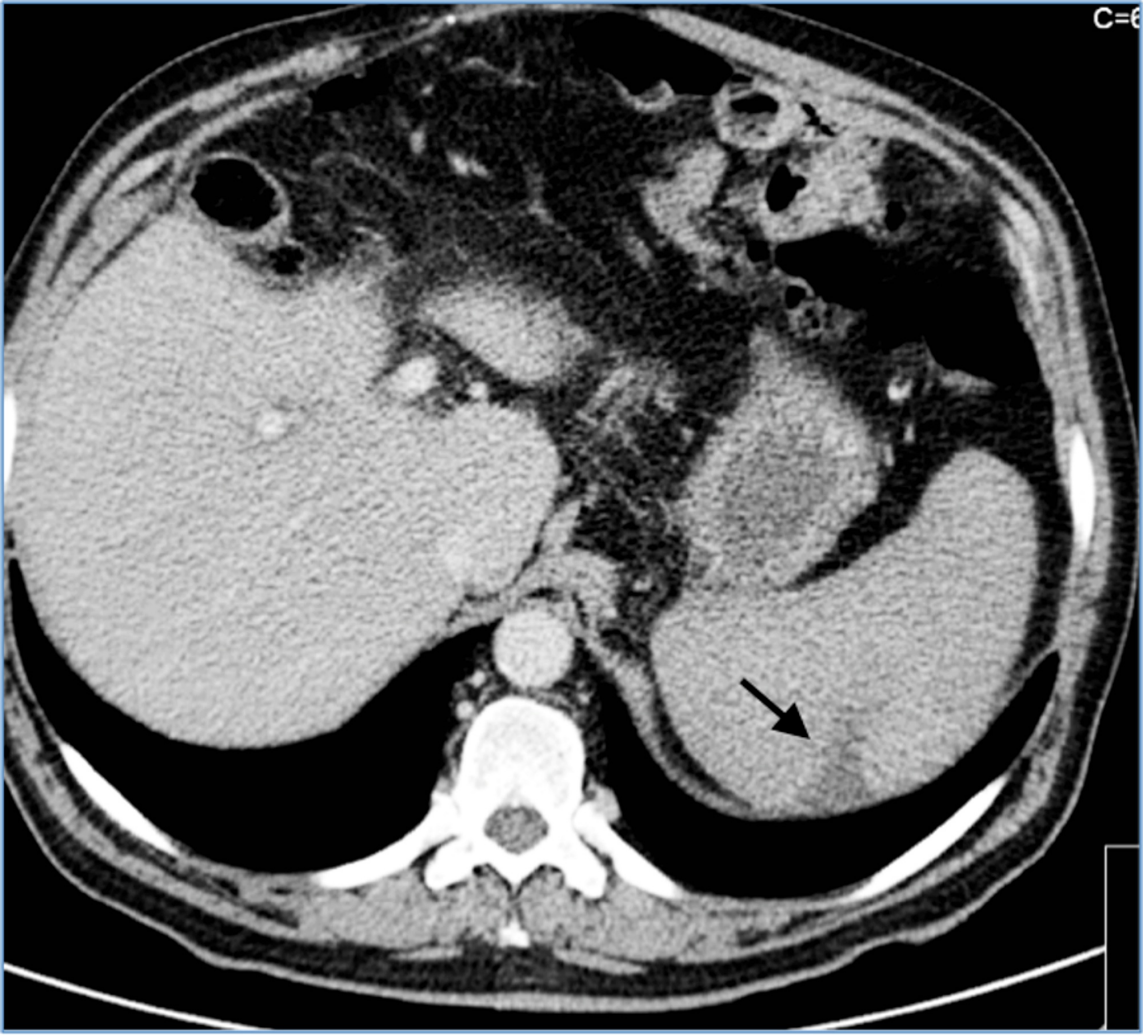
Abdominal CT scan showing an area of spleen infarct (black arrow)
